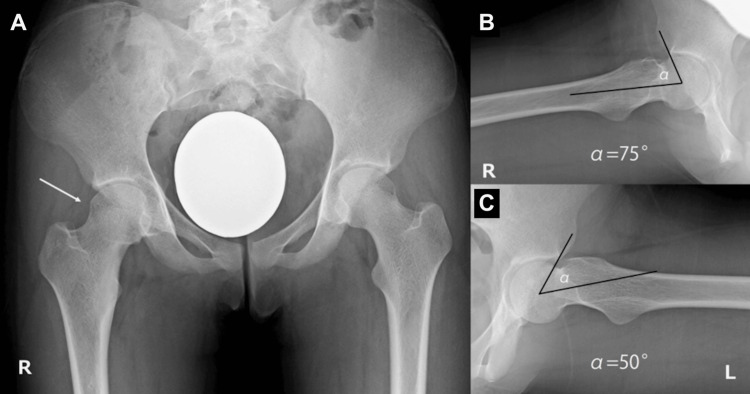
Preoperative anteroposterior (AP) and lateral radiograph of the hip joint (A) Bilateral cross-over signs and a sharp bony bump in the right femoral head-neck junction (arrow). (B) The alpha angles were 75° at the right hip and (C) 50° at the left hip.
